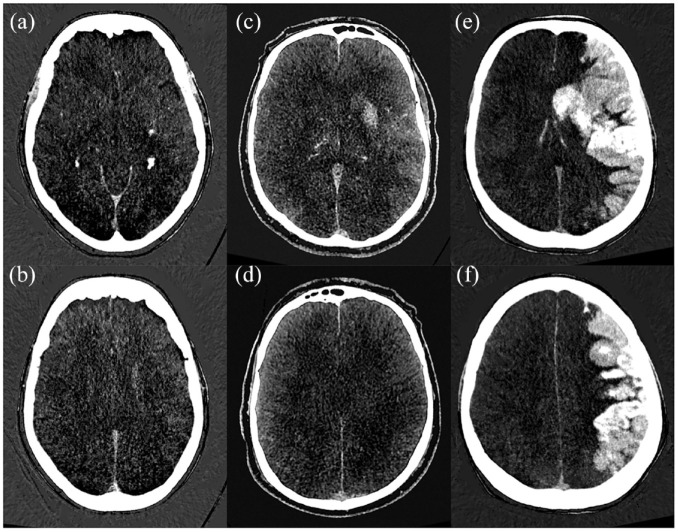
Contrast extravasation ASPECTS (CE-ASPECTS) on DECT iodine maps. In patient 1 (a and b), the caudate and lentiform nucleus show contrast extravasation (CE-ASPECTS = 8). In patient 2 (c and d), the caudate and lentiform nucleus, insula, M2 and M3 are affected (CE-ASPECTS = 5). In patient 3 (e and f), all areas but the posterior limb of the internal capsule are affected (CE-ASPECTS = 1).
